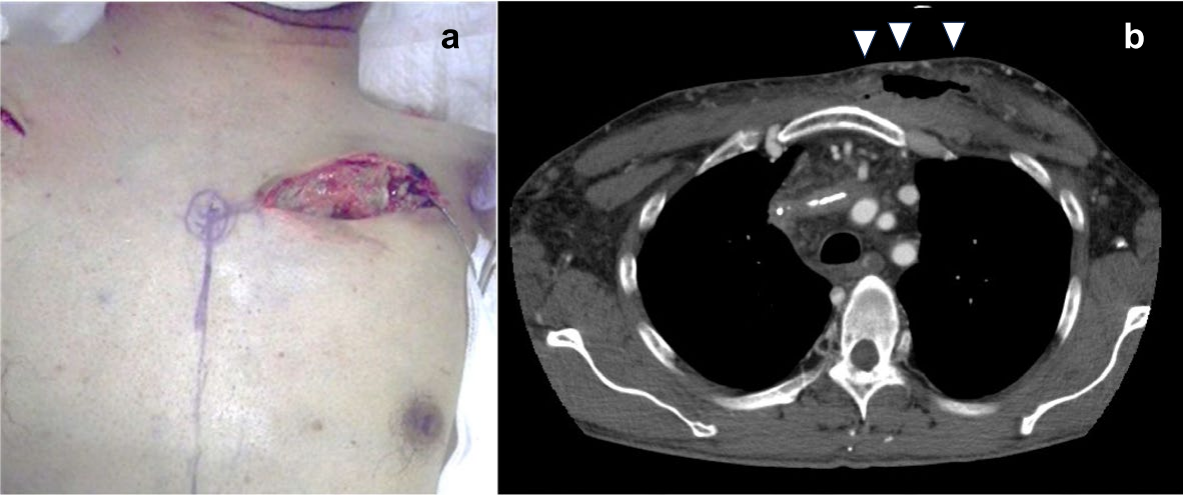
Open wound in the left anterior thorax (a) and chest CT (b). a The generator was removed and the RV lead remained. A subcutaneous pocket was formed in the midline and (b) extended to the anterior surface of the sternum (arrowheads)
